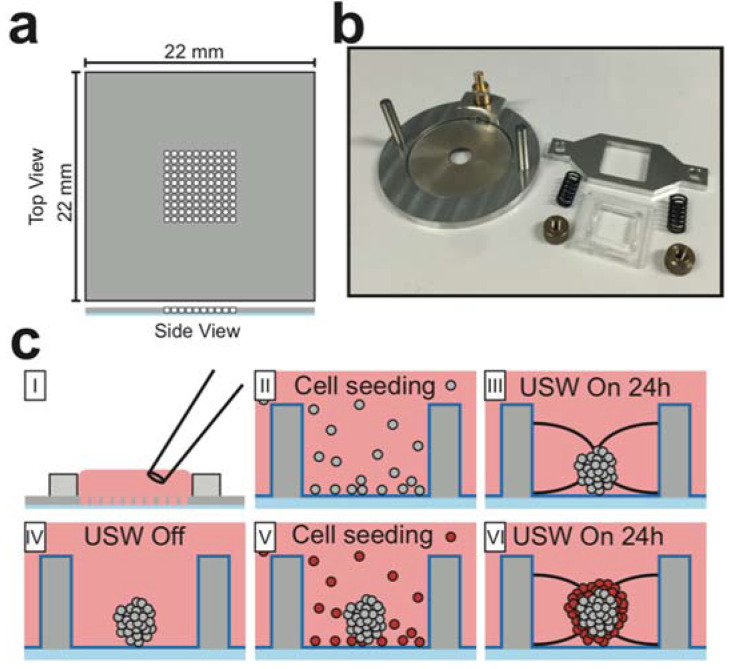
Ultrasound based layered multicellular tumor spheroids (MCTS) formation. The multi-well microplate (a) consists of a 22 × 22 × 0.3 mm^3^ silicon plate with 100 microwells (350 × 350 µm^2^) etched straight through the silicon layer and a 170 µm thick glass plate bonded to the bottom. The multiwell microplate was fixed on a circular transducer (b) with immersion oil as coupling medium to introduce ultrasonic standing waves (USWs) into the microwells. To form core-shell MCTSs (c), cells were seeded with a regular pipette into the shared medium reservoir above the microwells (I) and allowed to sediment to the well bottoms (II). The microplate was then transferred to the transducer which was actuated with a frequency corresponding to the λ/2-criterion across the microwell width. The radiation forces trapped all cells in an aggregate in the microwell center for 24 h (III). The microplate was dissembled from the transducer (IV) and cells comprising the shell were seeded around the preformed MCTS core (V) before the radiation forces were reintroduced for 24 h to create the core-shell MCTSs (VI).
